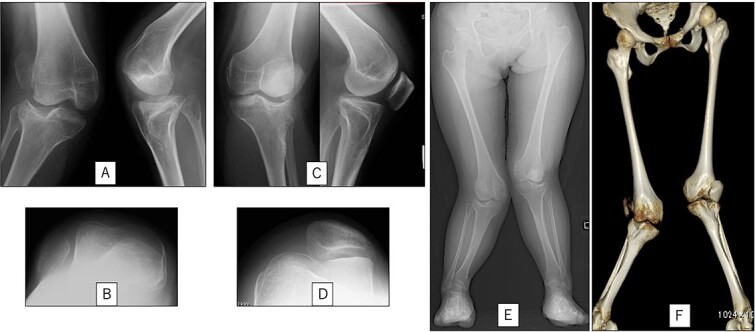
Preoperative radiography and computed tomography: (A) anteroposterior and lateral views of the right knee, (B) skyline view of the right knee, (C) anteroposterior and lateral views of the left knee, (D) skyline view of the left knee, (E) standing anteroposterior view, and (F) 3D image of the entire lower limb.
